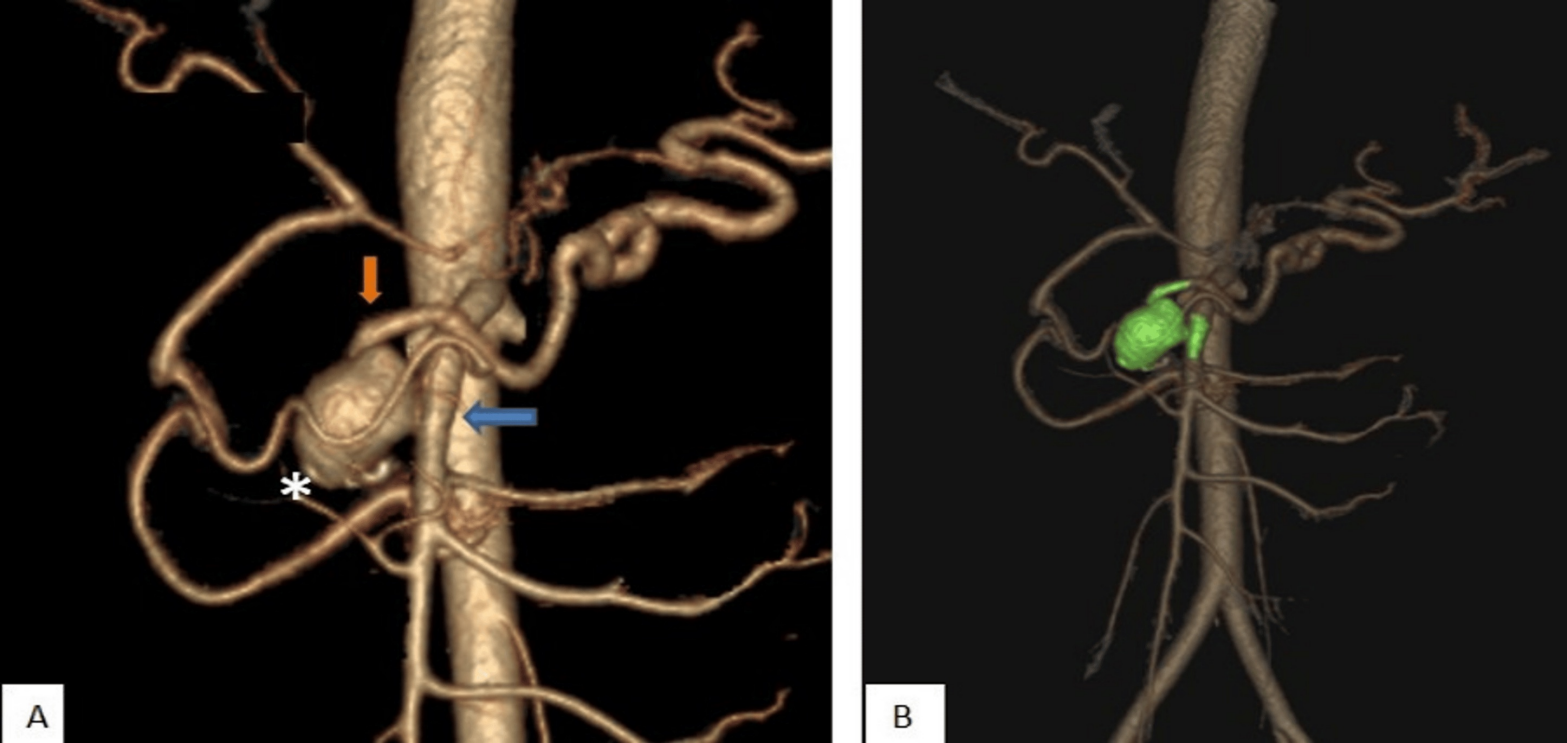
CECT volume-rendered imaging (Case 1) (A) AOB aneurysm (asterisk) is seen in close relation with the SMA (blue arrow) with a collateral branch (orange arrow) arising from its postero-lateral aspect, seen to continue as the splenic artery (a branch of celiac artery); (B) Arch of Buhler is seen between the SMA and the suspected celiac bed with an aneurysm originating from this abnormal artery CECT: contrast-enhanced computed tomography; AOB: Arc of Buhler; SMA: superior mesenteric artery
